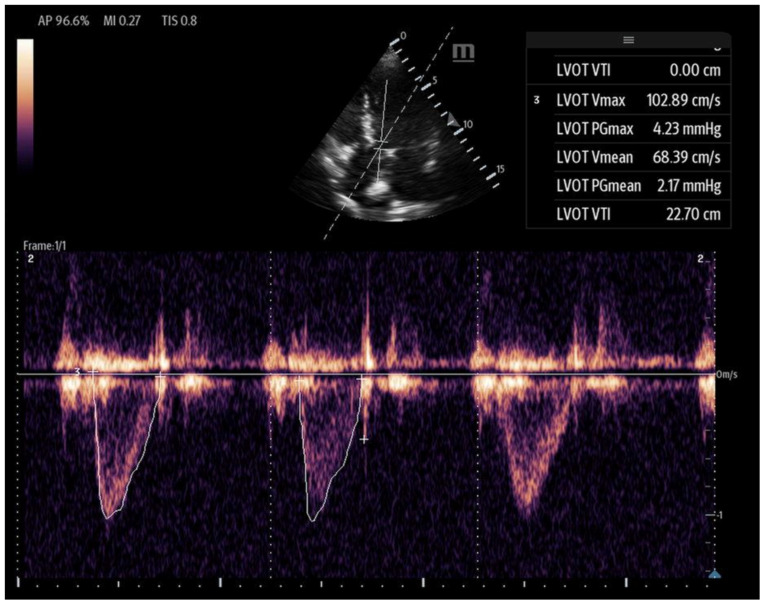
Left ventricular VTI calculated with PW Doppler with Doppler gate at LVOT.
